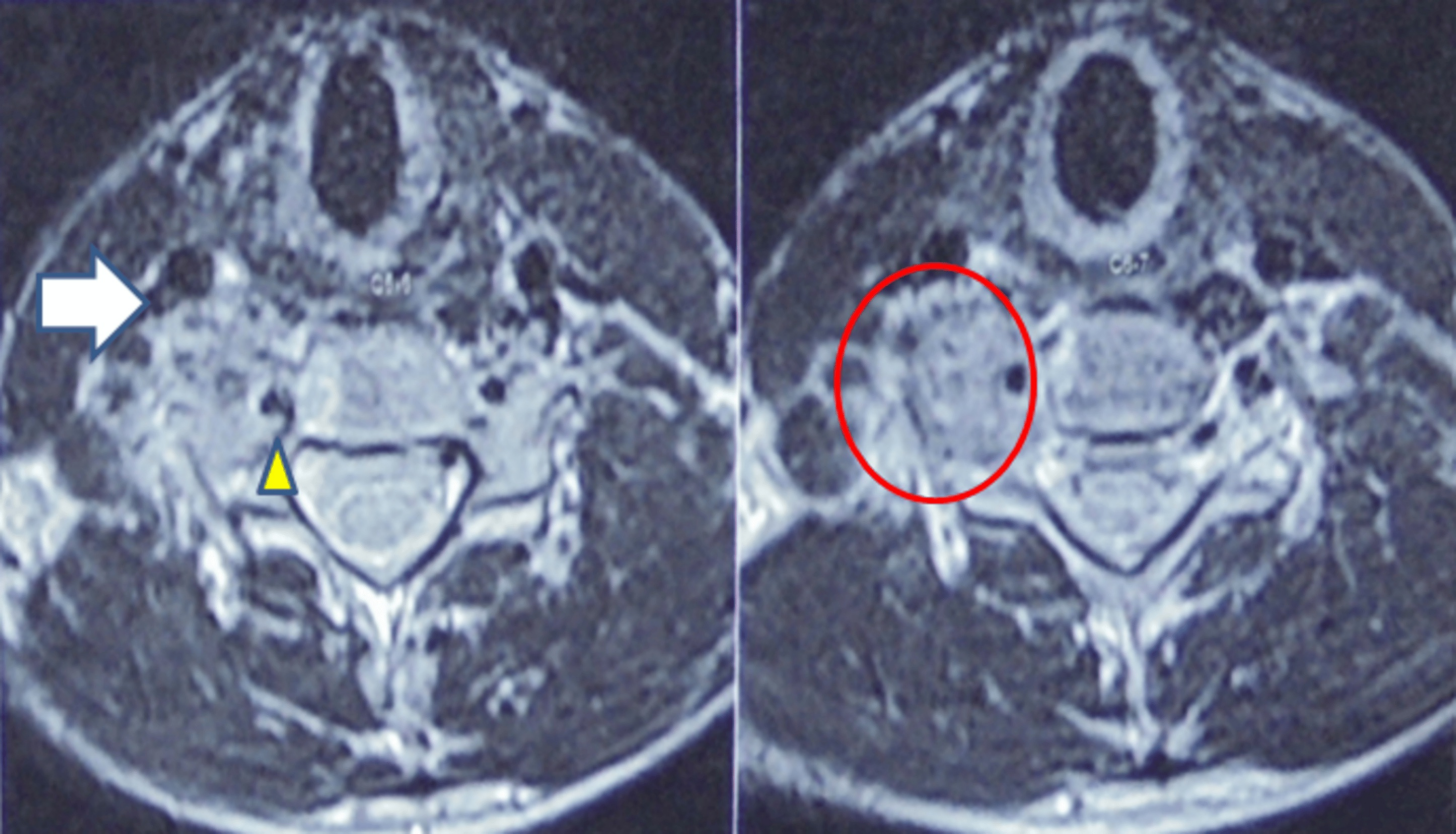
MRI of the cervical spine demonstrating tumor characteristics. The sequence shows heterogeneous intermediate to high intensity on T2-weighted images, consistent with a vascularized, mineralized lesion, which is marked in the circle. The arrow points to the proximity of the tumor to the right carotid artery, and an arrowhead points toward the right vertebral artery MRI: magnetic resonance imaging
